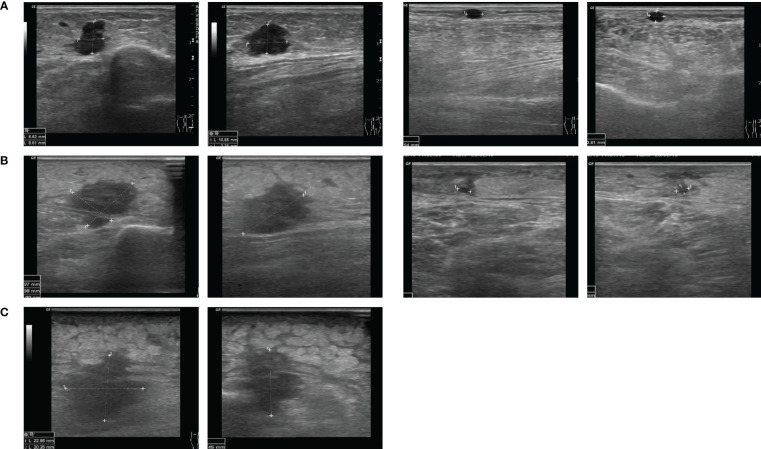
Course of subcutaneous melanoma metastases under combination of MEK inhibitor and T-VEC in case 3. Ultrasound images from (A) August 2018, (B) February 2019 and (C) July 2019.
